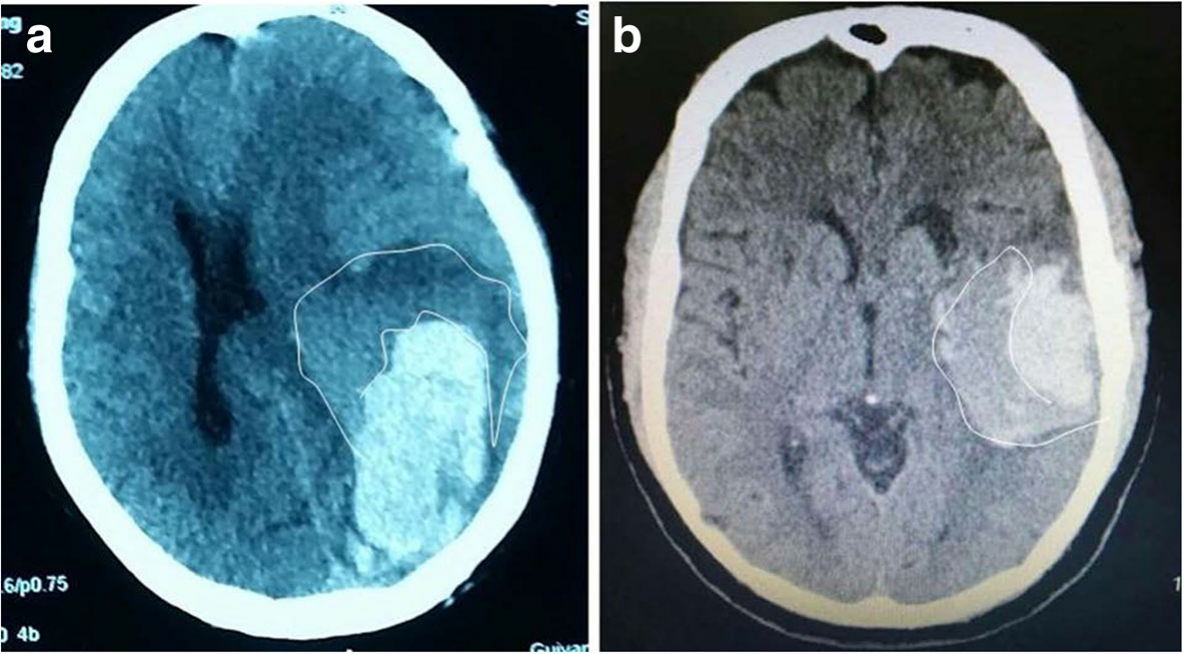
Blend sign on initial CT scan. The blend sign consists of two parts with apparently different CT attenuations. a The blend sign in patients with cerebral lobe haemorrhage. b The blend sign in patients with basal ganglion haemorrhage
